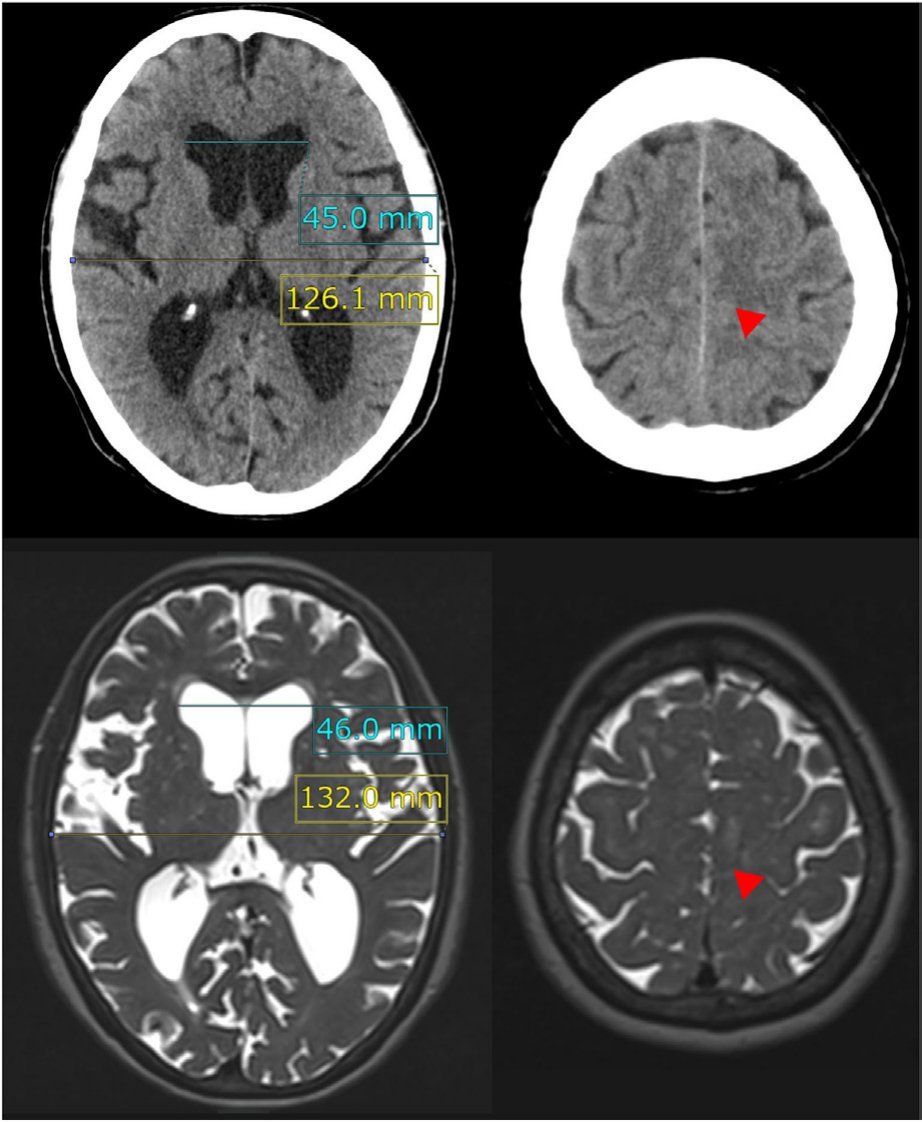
Evans index and high-convexity tightness. The upper figures show the traditional axial planes on CT scan and the lower figures show the T2-weighted MRI in a representative case of idiopathic NPH. The Evans index is measured as the maximal width of the frontal horns of the bilateral ventricles (sky blue line) to the maximal width of the internal diameter of the cranium (yellow line) on the basis of the axial planes on CT or MRI. In this case, the Evans index was calculated as 0.36 on CT scan and 0.35 on MRI. Red arrowheads indicate the high-convexity tightness which means decreased subarachnoid spaces at the convexity.
